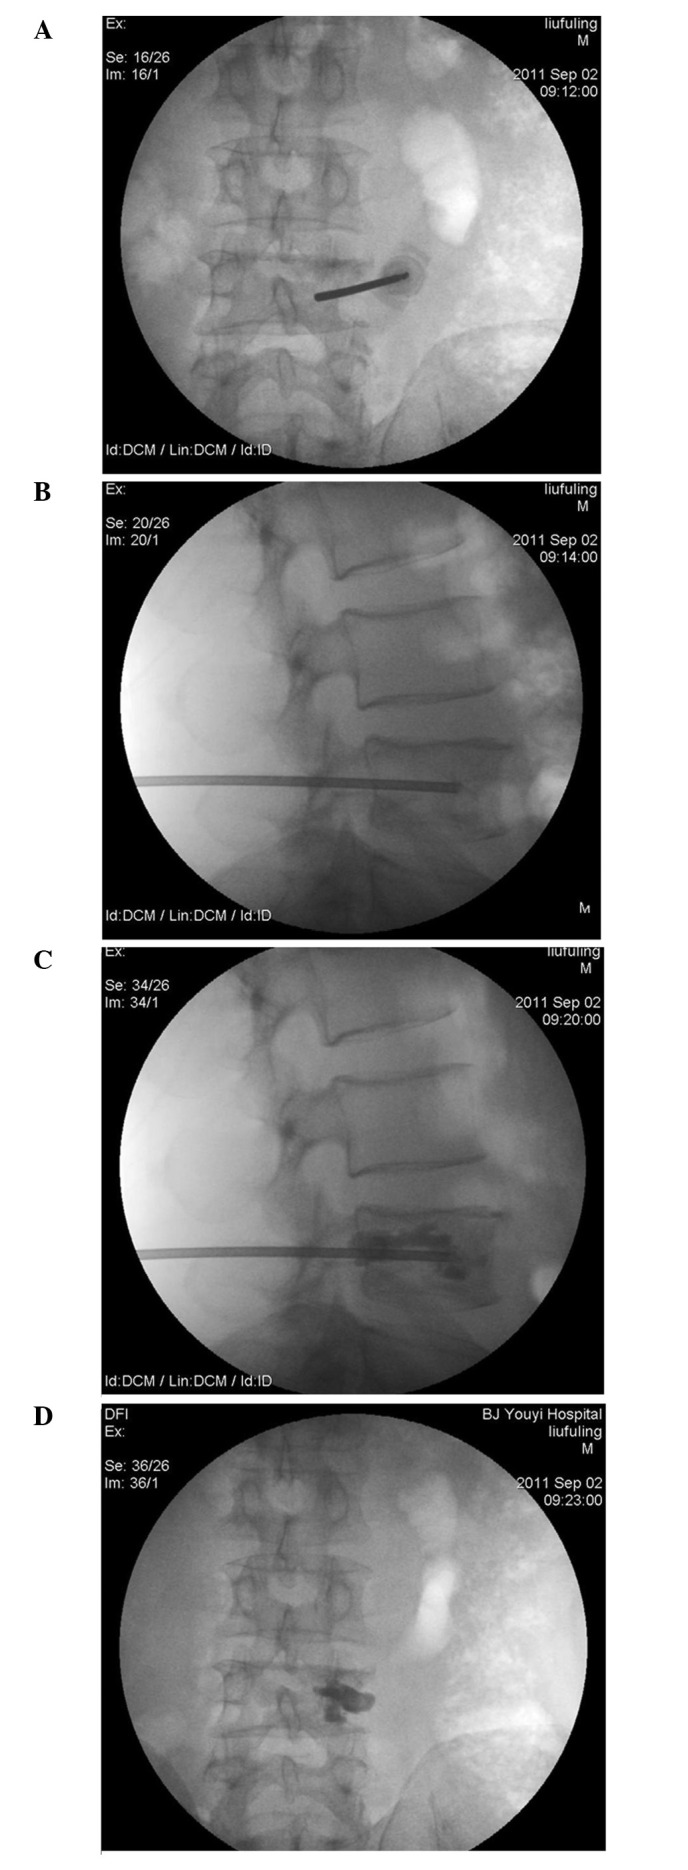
Radiography during PVP of the L4. Right posterolateral approach. Injection of polymethylacrylate and penetration of the L4. Images show cement filling at the right side of the vertebral body. PVP, percutaneous vertebroplasty; L4, fourth lower lumbar vertebra.
